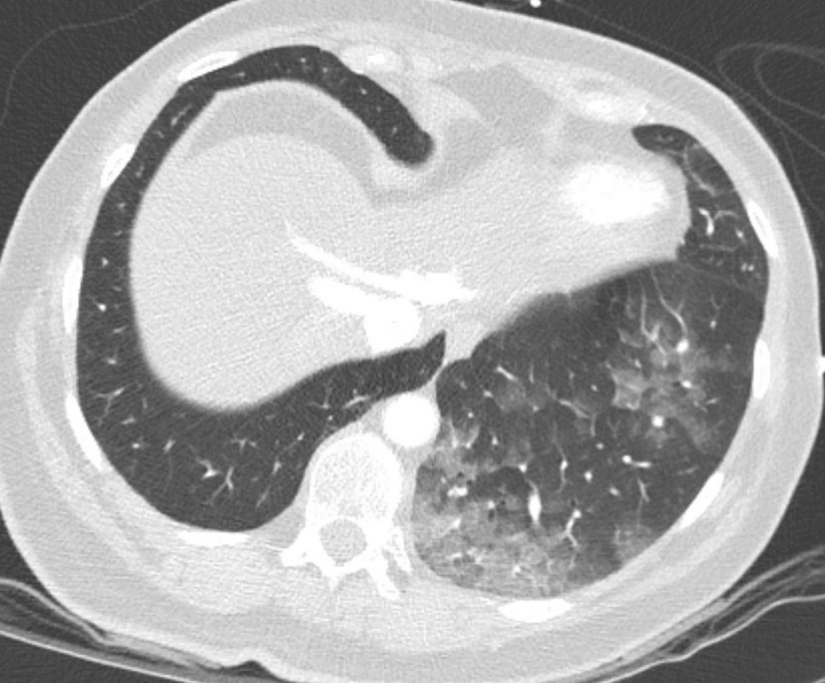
Axial CT image shows ground-glass opacities in the right upper lobe, together with smooth interlobular septal thickening without pleural effusion in the absence of other typical CT findings compatible with COVID-19, classified as CORADS 3
